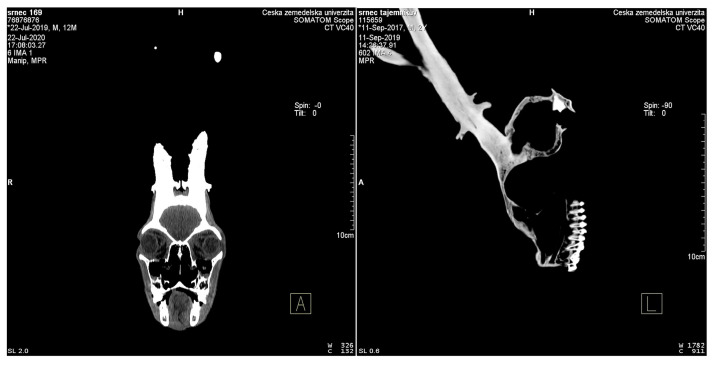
CT scanner—an internal image of a roe deer skull with visible tooth roots and antler structure.
